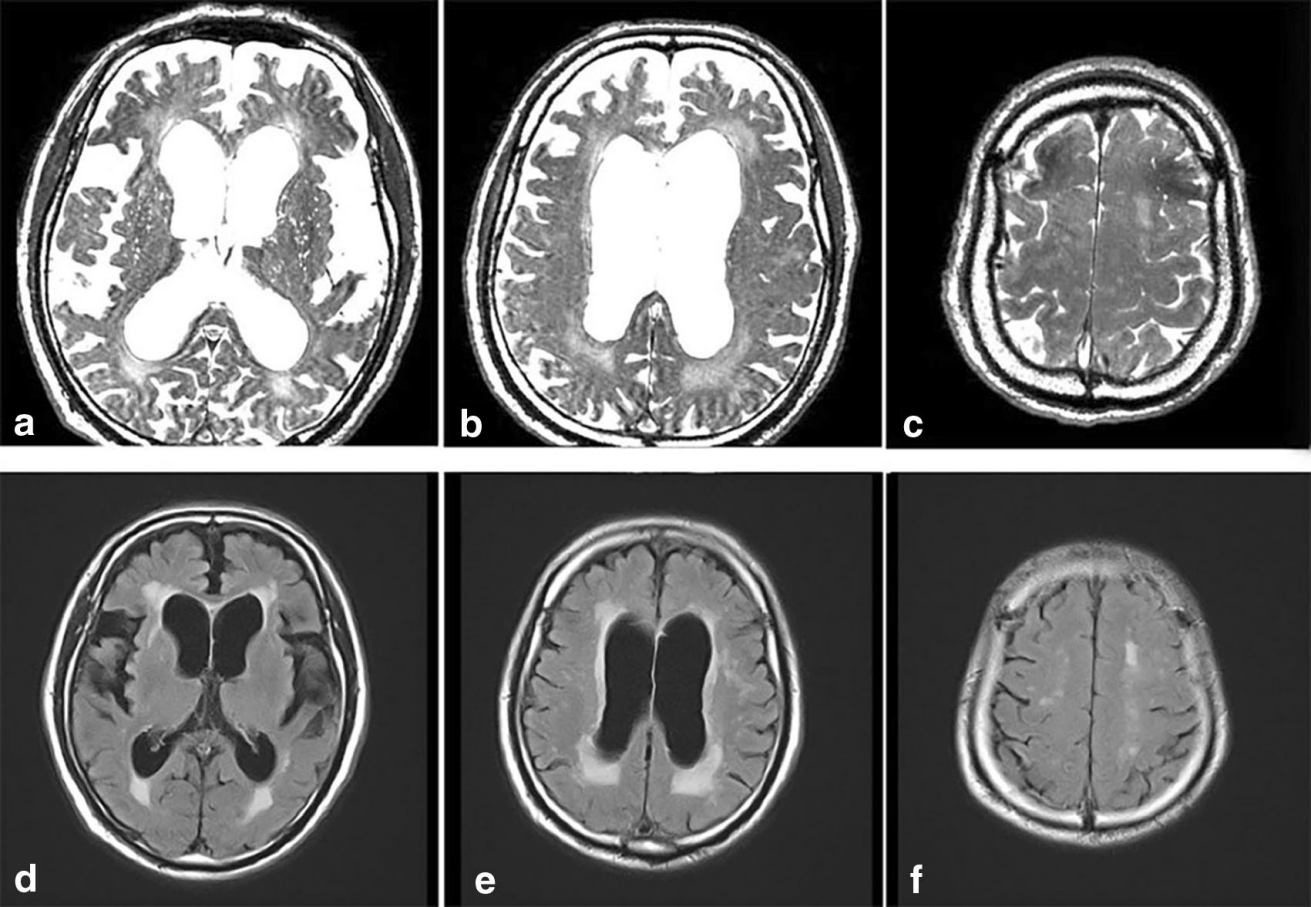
Virchow–Robin spaces (VRS) in an 82-year-old patient with idiopathic normal pressure hydrocephalus (iNPH). In iNPH, constructive interference in the steady state images (a–c) showed few VRS in the white matter, in contrast to abundant VRS in the basal ganglia. A moderate degree of hyperintensities was noted in the periventricular and semioval center white matter. Corresponding images of fluid attenuated inversion recovery (FLAIR) are shown in d–f.
